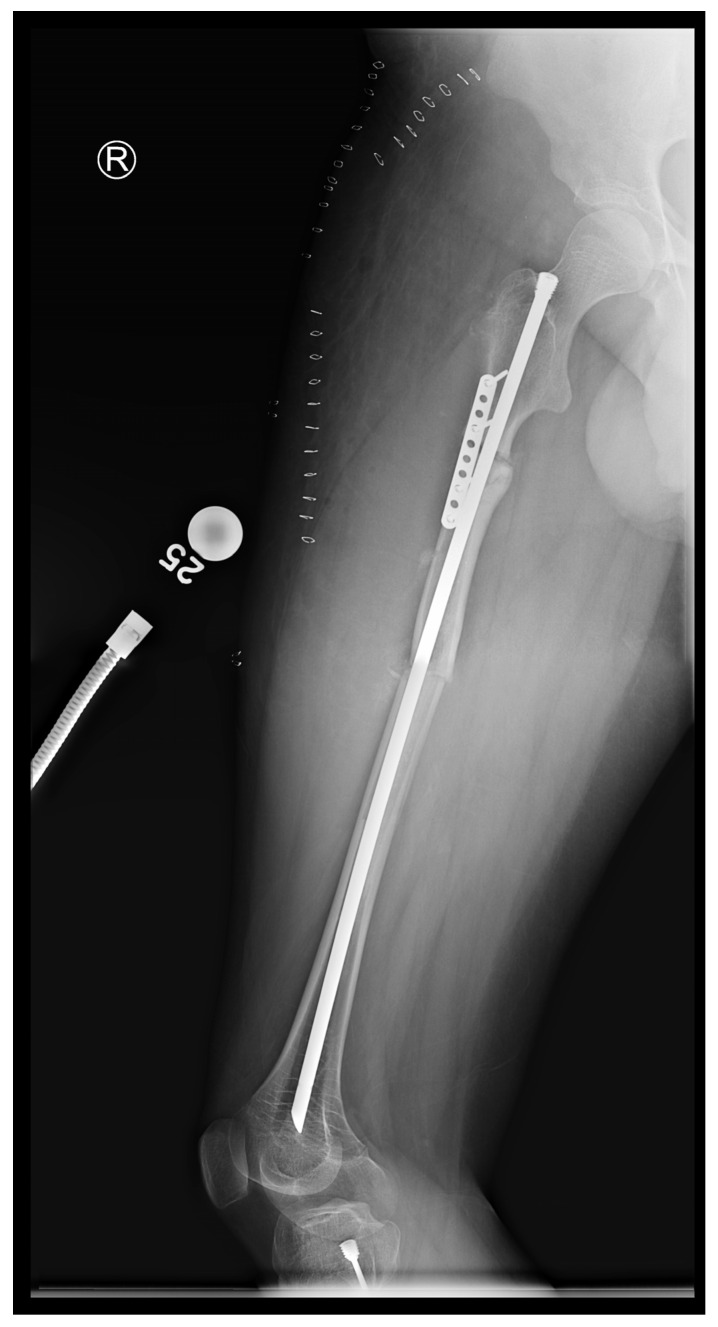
Post-operative X-ray showing SLIM nail and plate.
